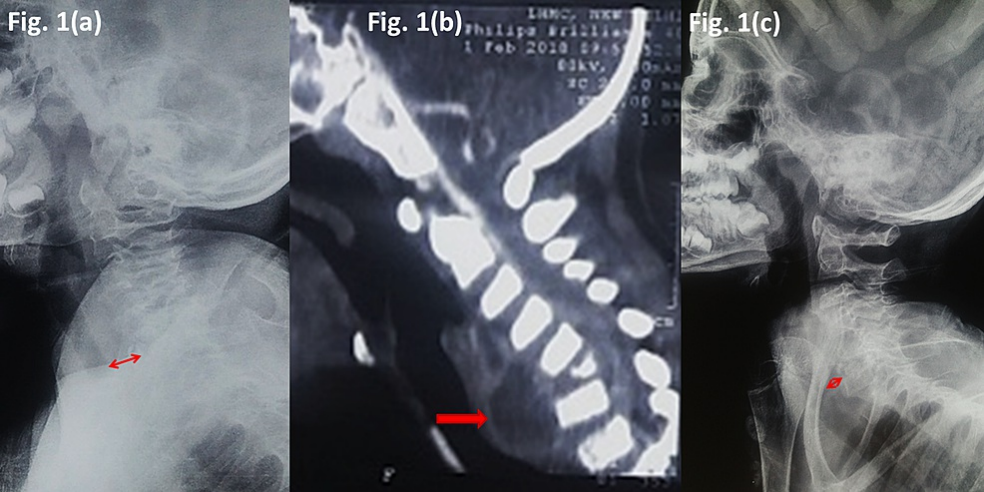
Cervical spine TB with cerebral palsy (a) Plain radiograph at presentation (b) CT scan (c) After 12 months of ATT TB - tuberculosis, ATT - anti-tubercular drug therapy
